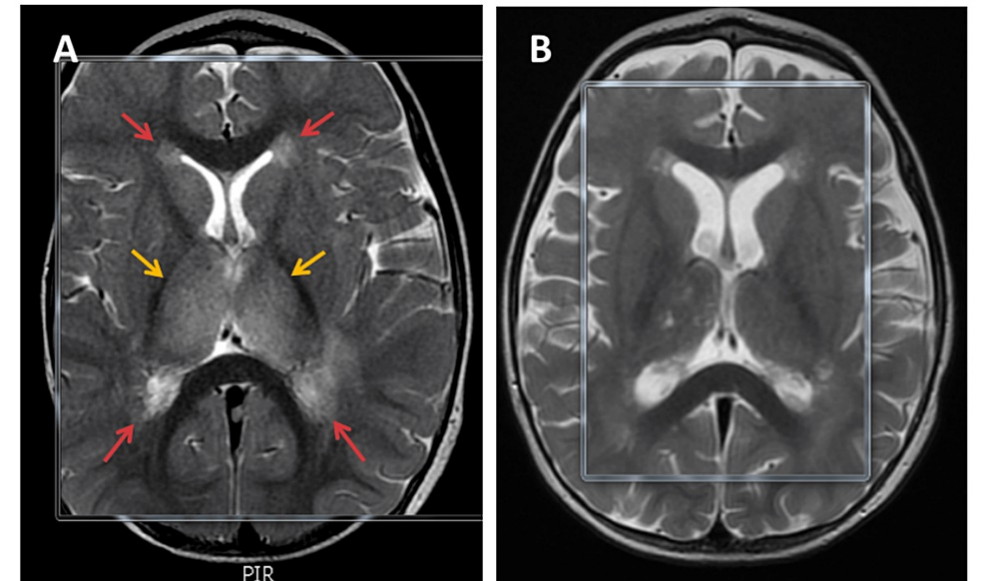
The axial image of the T2 modality The axial image of the T2 modality (A) demonstrates a high signal intensity in the bilateral periventricular area (red arrows) with swollen hyperintensive thalami (yellow arrows), which is a characteristic finding of acute necrotizing encephalopathy of childhood. In the repeated brain MRI images, there was an interval regression of T2 modality hyperintensity in bilateral deep periventricular white matter, with regression of swelling of the thalami and temporal lobes (B). Also, there is a new development of enhancement in bilateral symmetrical anterior temporal horns and lateral ventricles. These areas showed diffusion restriction in the previous study, which likely represents infarcts in the late subacute stage.
